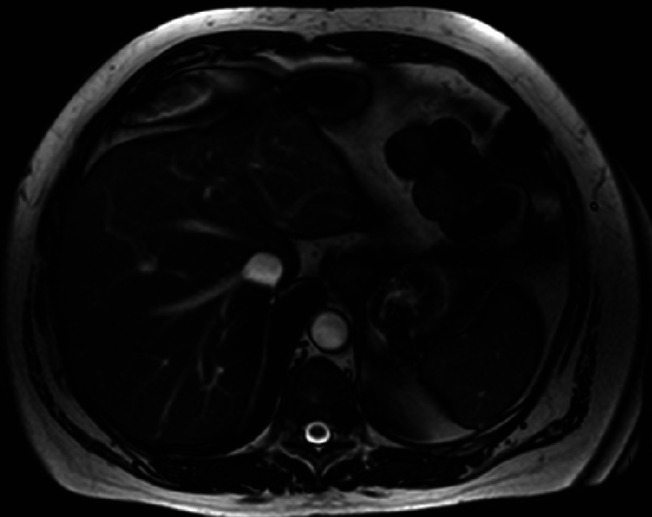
T2-weighted imaging of the liver posttreatment of *M. fortuitum.*
